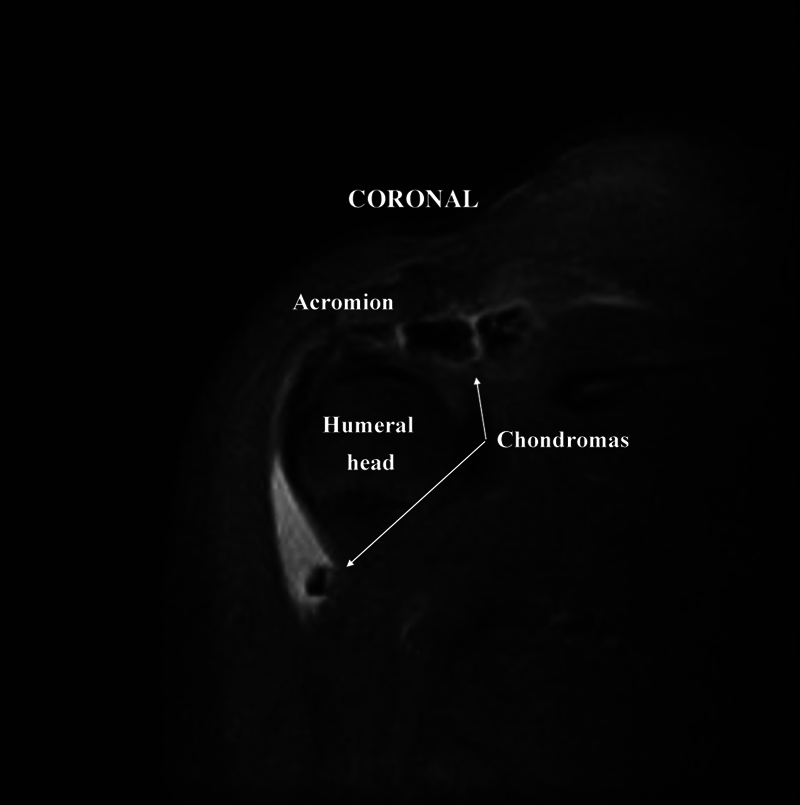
Magnetic resonance imaging of the shoulder (coronal view, T2-weighted image).
